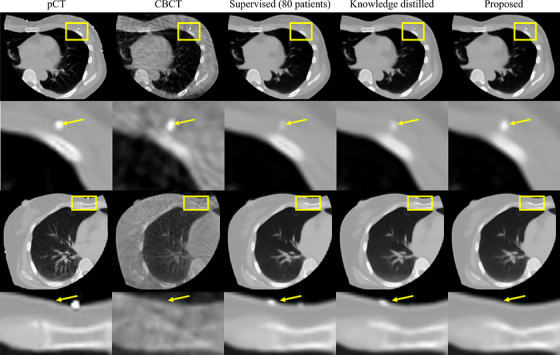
Qualitative comparison of the progressive training stages. The intermediate Supervised and Knowledge‐distilled models demonstrate a tendency to hallucinate features from the pCT, such as the fiducial marker (bottom row, yellow arrow). In contrast, our final Proposed model shows superior anatomical fidelity by enhancing image quality while correctly preserving the true anatomical structure of the daily CBCT, as evidenced by the well‐preserved clip structure (top row). All images are displayed with a window setting of [‐1000, 700] HU.
